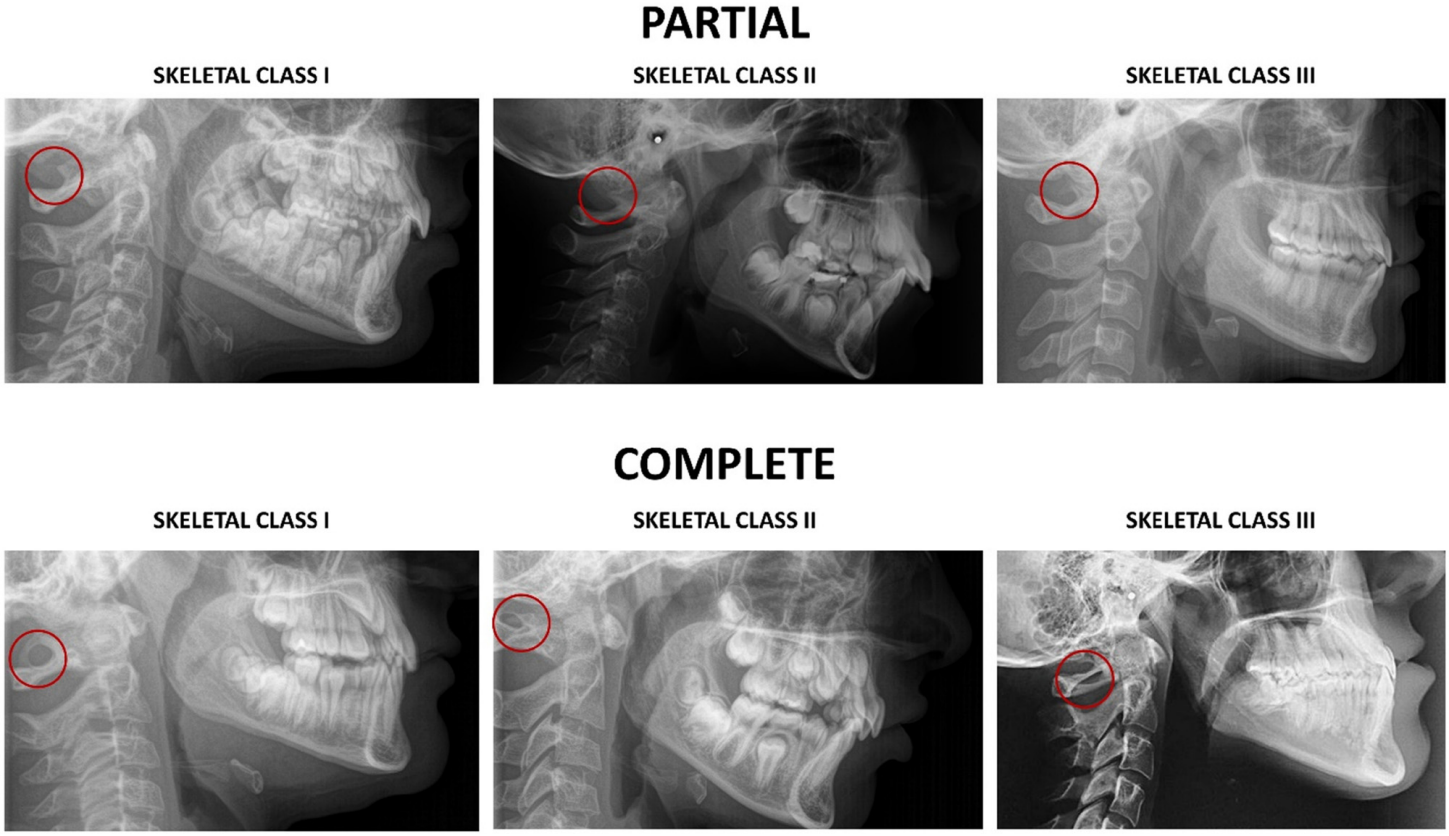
Radiographic representation of the complete or incomplete PP presence divided by the Angle’s classification of malocclusion (I, II, or III).
